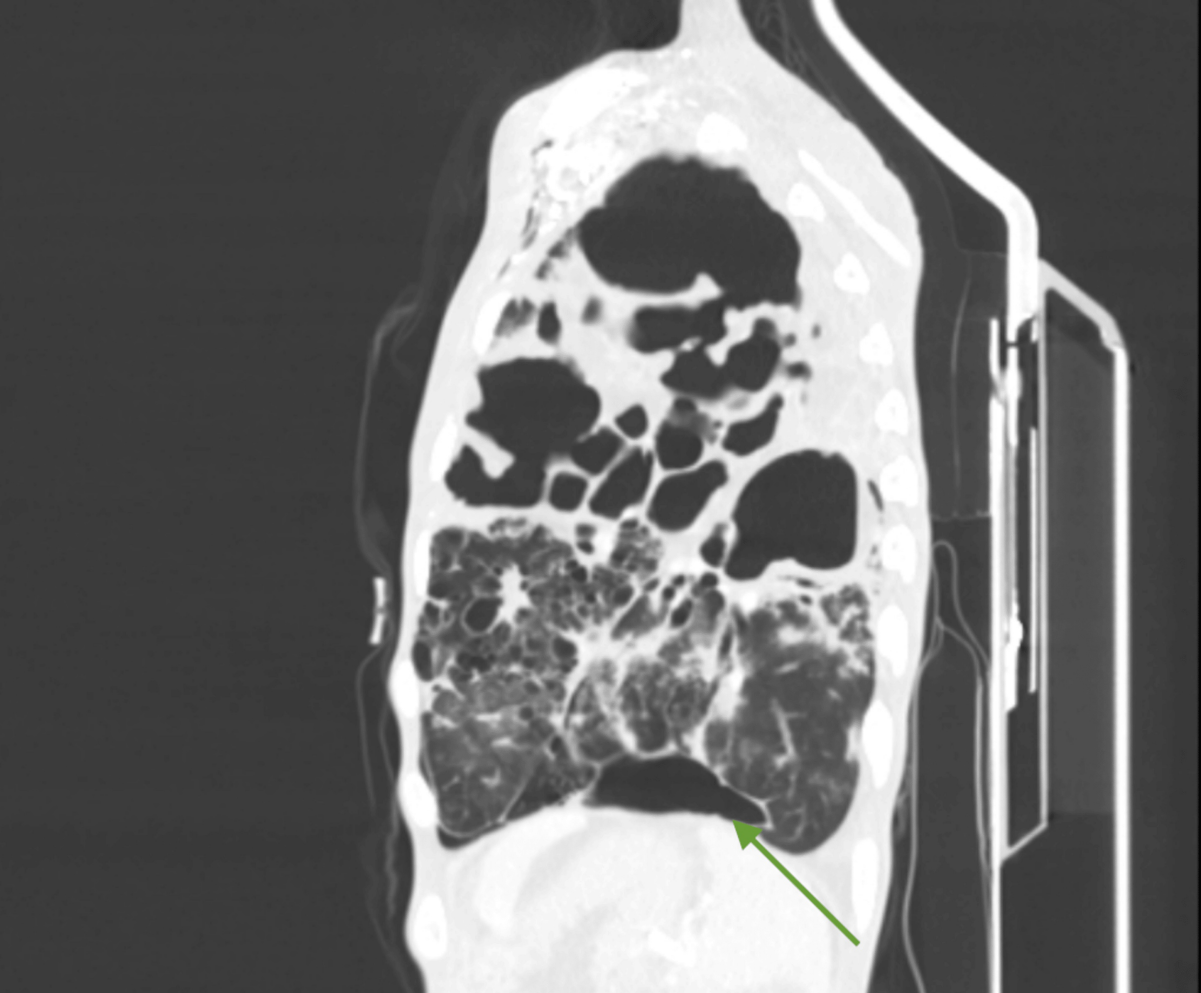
Sagital plane of the left hemitorax on the contrast enhanced CT Showing another perspective of the largest cavitations. Green arrow: the small pneumothorax between the diaphragm and the pericardium.
